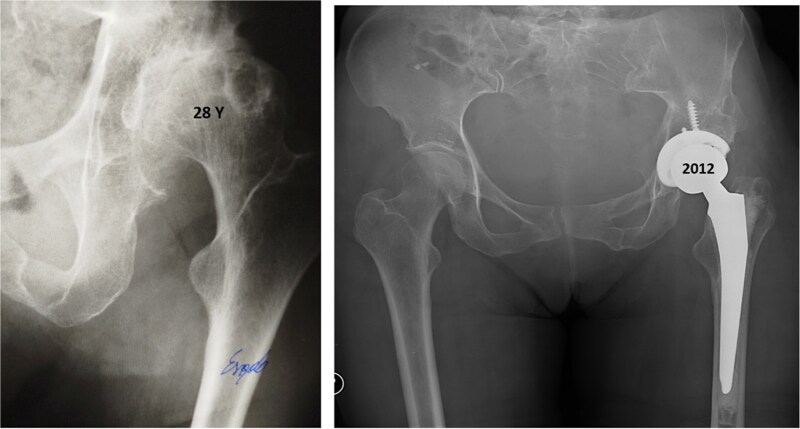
Anteroposterior radiographs of the pelvis 28 years after the pelvic osteotomy, showing advanced left hip osteoarthritis (Tönnis Grade V). A hybrid cemented total hip arthroplasty was implanted using a standard posterolateral approach.
